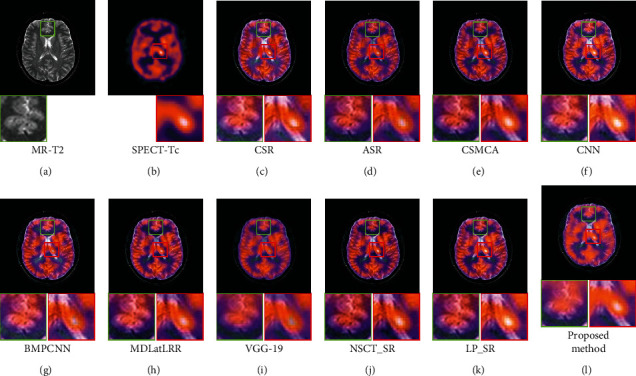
Comparison of the fusion results in the fourth set images. (a) MR-T2. (b) SPECT-Tc. (c) CSR. (d) ASR. (e) CSMCA. (f) CNN. (g) BMPCNN. (h) MDLatLRR. (i) VGG-19. (j) NSCT_SR. (k) LP_SR. (l) Proposed method.
